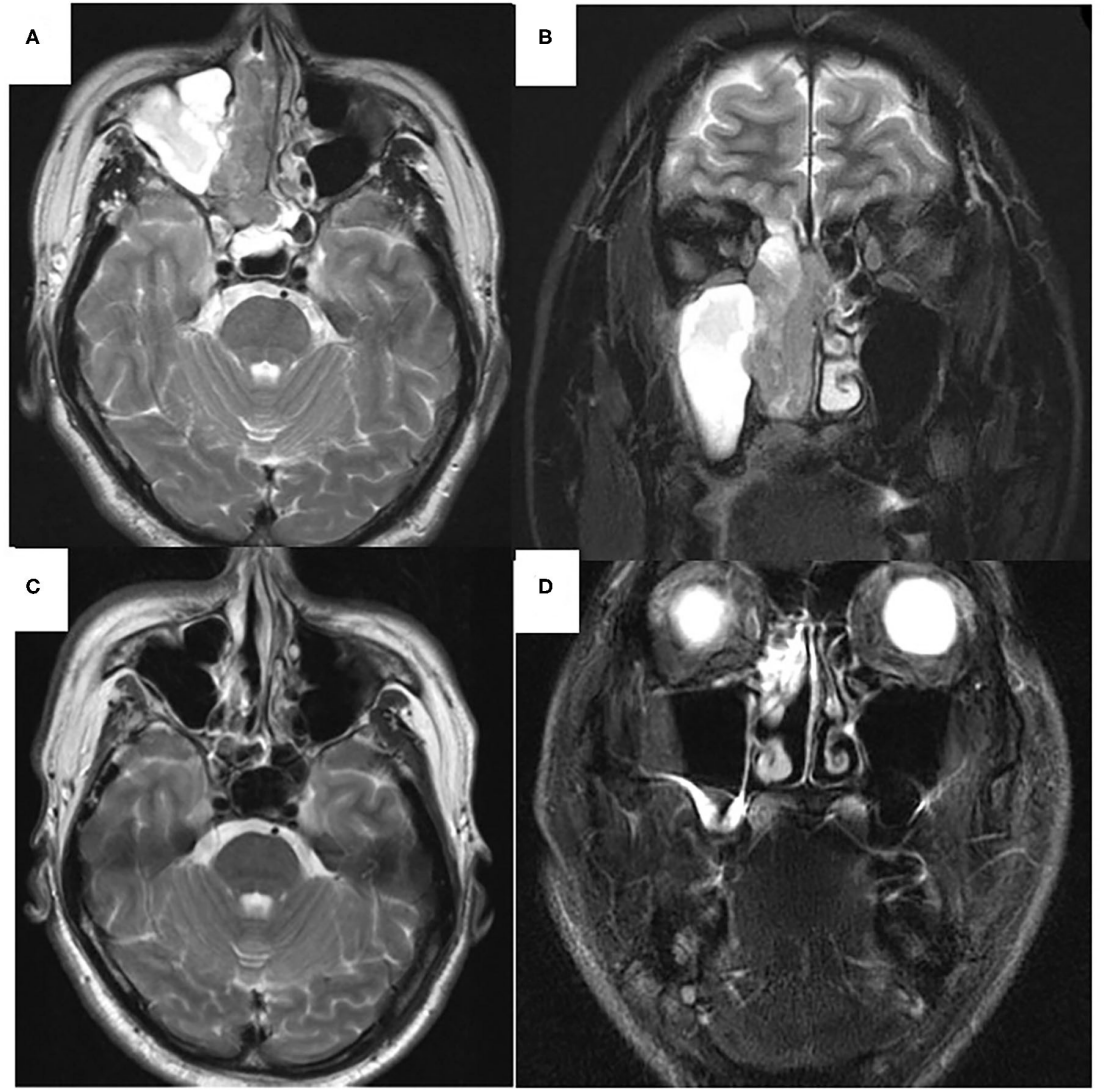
T2-weighted magnetic resonance imaging (MRI) of the sinonasal region. Axial view (A,C). Coronal view (B,D). Before chemoradiotherapy (A,B). Low signal area was observed mainly in the right nasal cavity, ethmoid and sphenoid sinuses. High signal intensity area was found in right maxillary and left sphenoid sinuses. After chemoradiotherapy (C,D). In right maxillary sinus, high signal intensity area was disappeared (C). High signal intensity area was observed within the ethmoid sinus (D).
